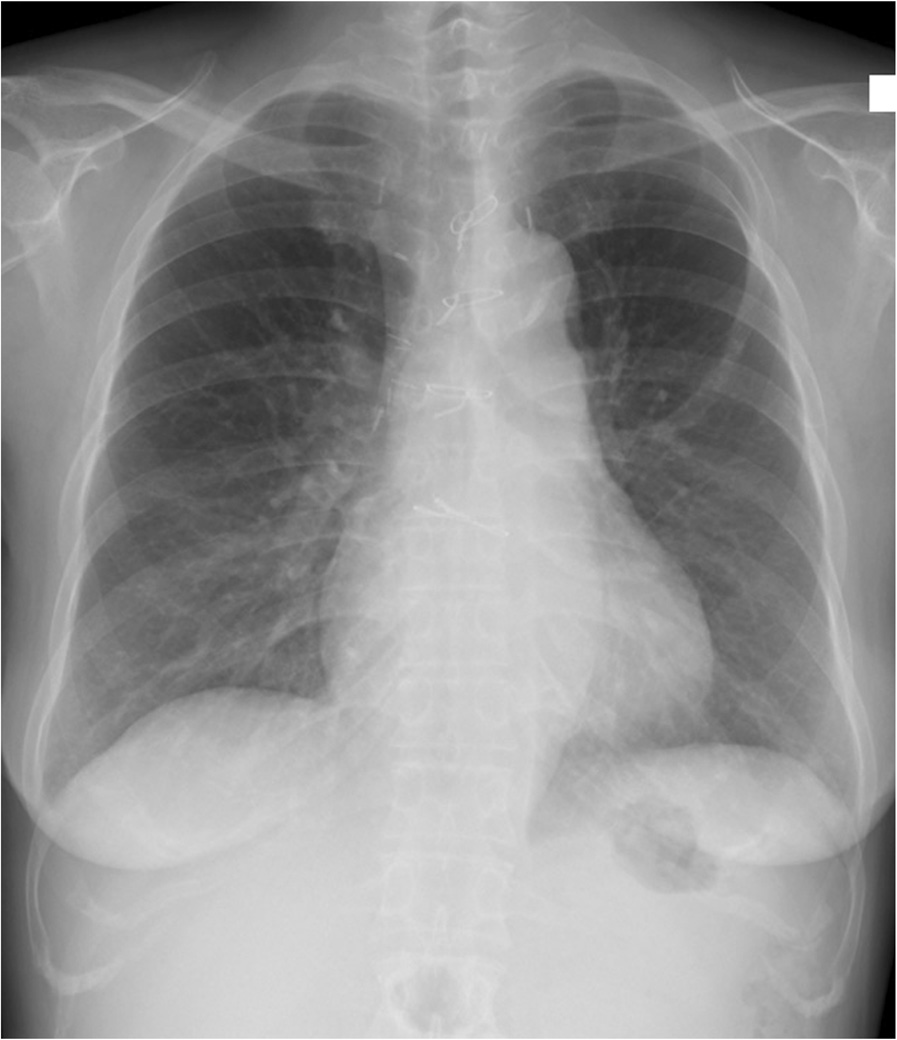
Chest roentgenogram obtained 3 months after surgery
